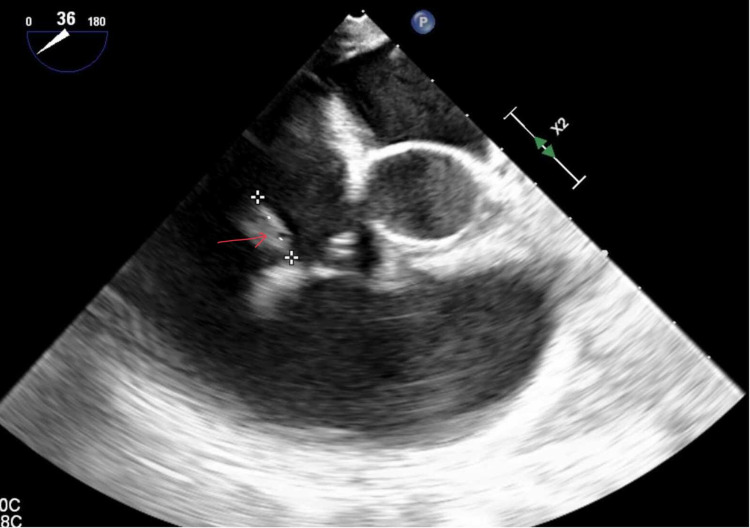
TEE demonstrates reduction of vegetations on the anterior and the septal leaflet TEE: transesophageal echocardiography
